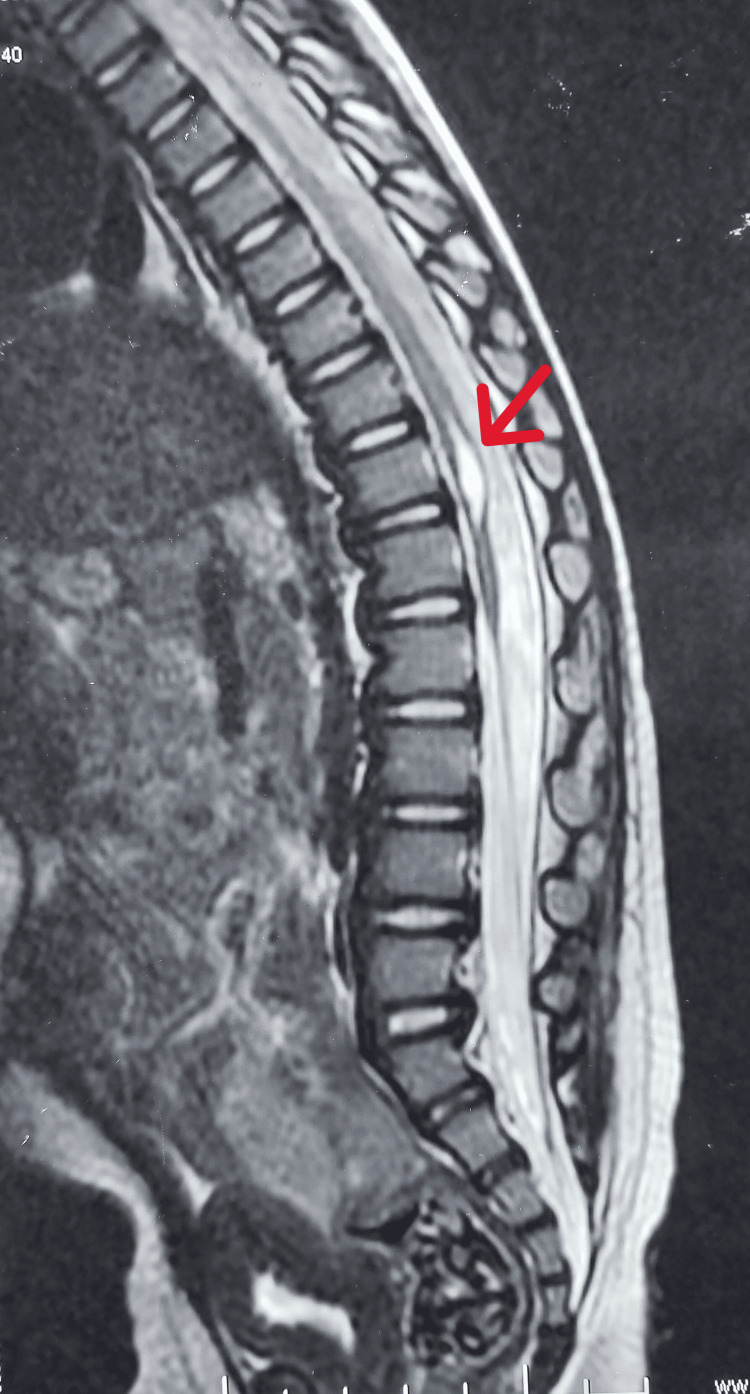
MRI of the spine showing the presence of a short segment syrinx from D10 to D12 level (indicated by an arrow).
